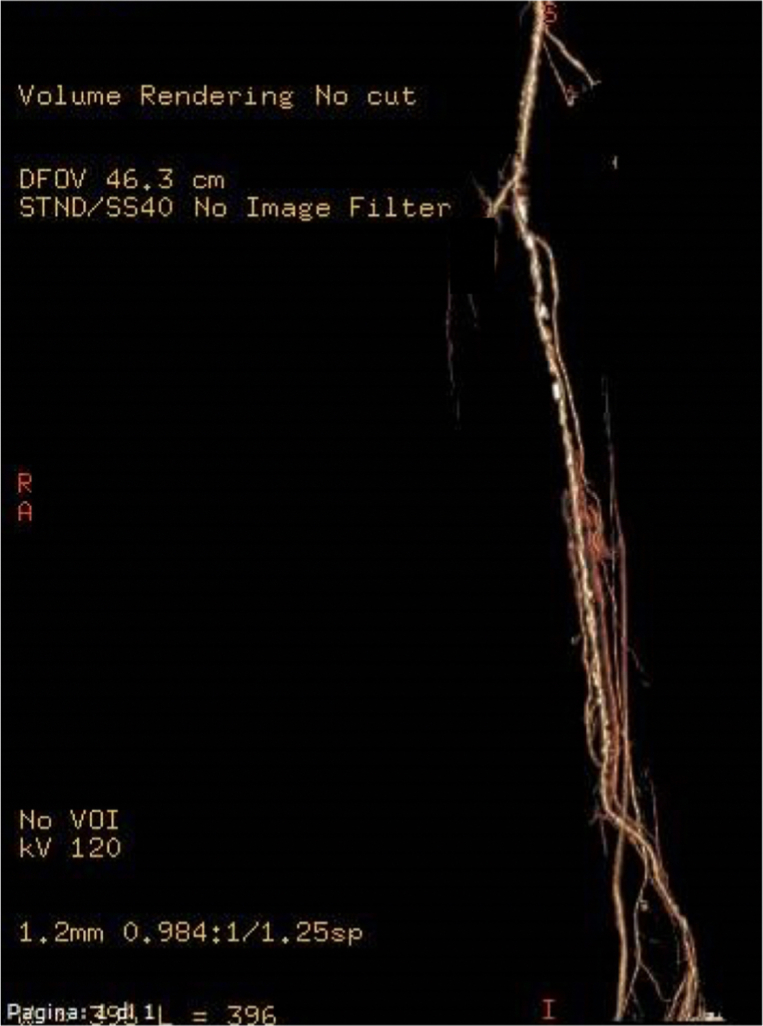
Computed tomography scan at 3 months of follow-up.
